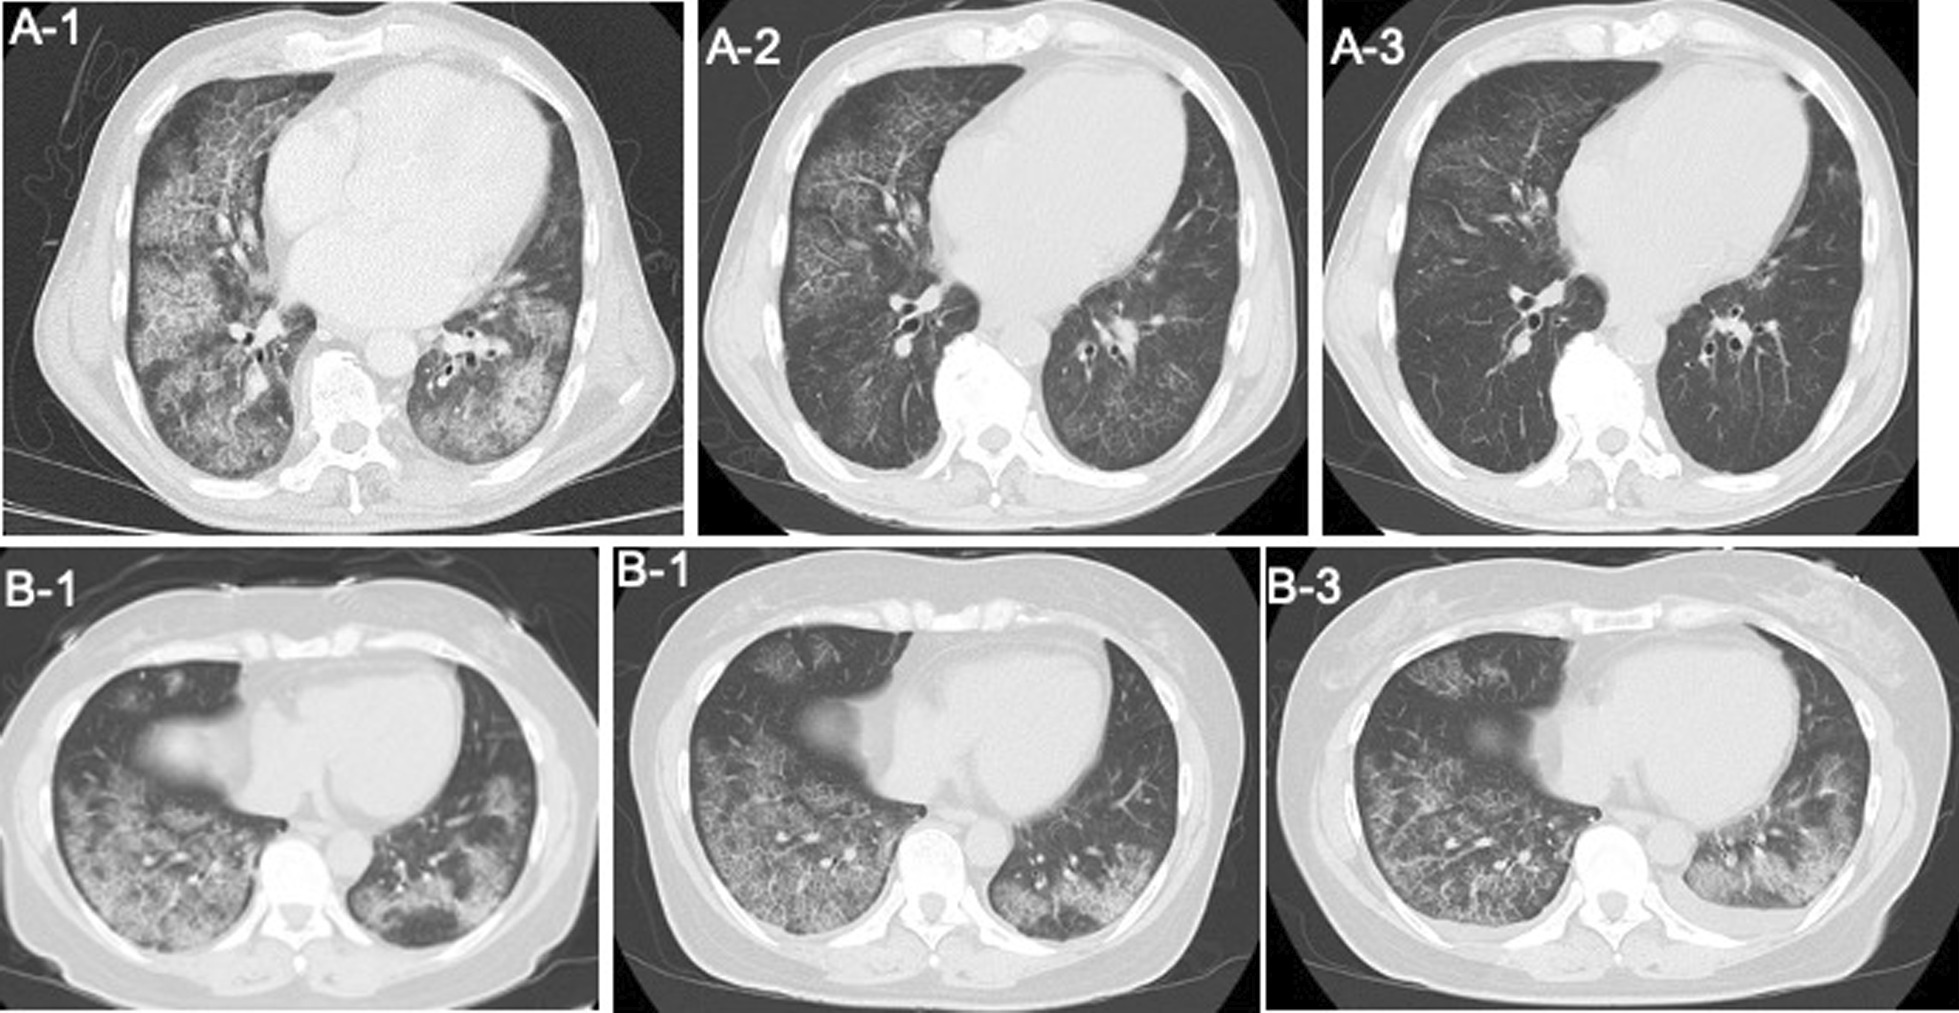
Chest computed tomography and sample collection. Chest computed tomography in A Case 1 and B Case 2. A-1, B-1 Before WLL: A crazy-paving pattern is observed in both cases. A-2 At 1 week after left lung lavage. B-2 At 3 days after right lung lavage. A-3 At 1 week after right lung lavage. B-3 At 4 days after left lung lavage
